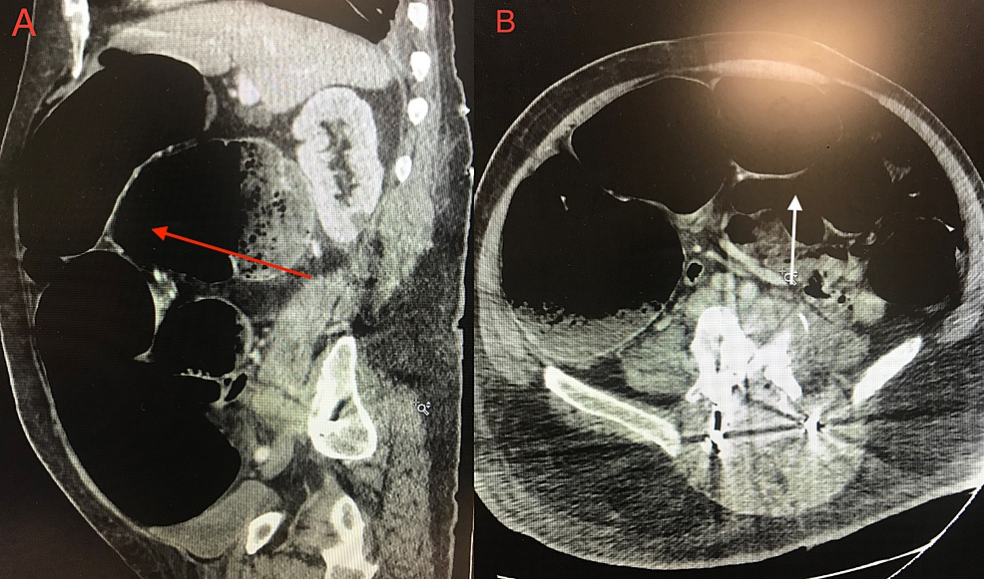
(A) Sagittal view of CT abdomen showing acute colonic dilation shown by the red arrow. (B) Coronal view of CT abdomen showing acute colonic dilation shown by the white arrow.
